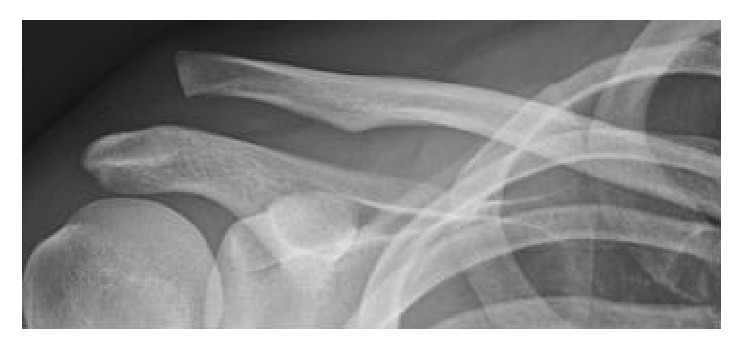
Radiograph of a 24-year-old male patient after a cycling accident; dislocation of the AC joint (Rockwood V).
